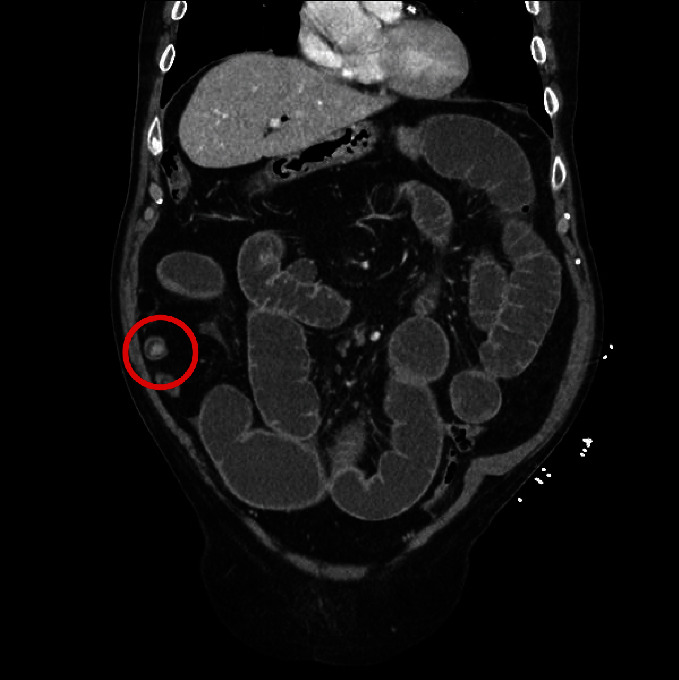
CT imaging demonstrating small bowel obstruction and gallstone impacted in the distal ileum.
